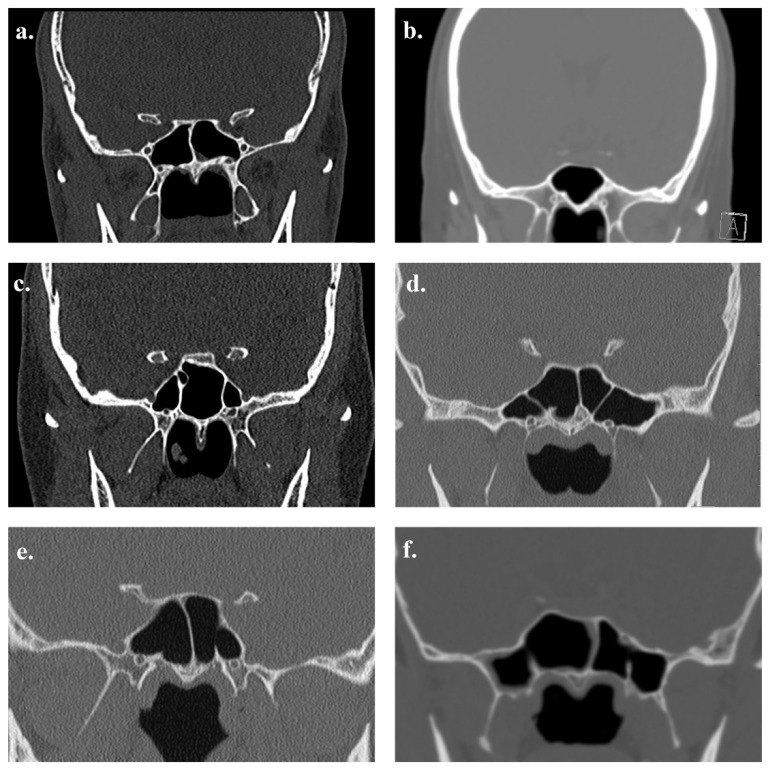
Coronal CT images demonstrating variations in sphenoid inter-sinus septation: (a) single central complete septum; (b) absent septum; (c) two complete septa; (d) three complete septa; (e) two septa, consisting of a central complete septum and a left-sided incomplete septum; and (f) three septa, consisting of a central complete septum with right- and left-sided incomplete septa.
